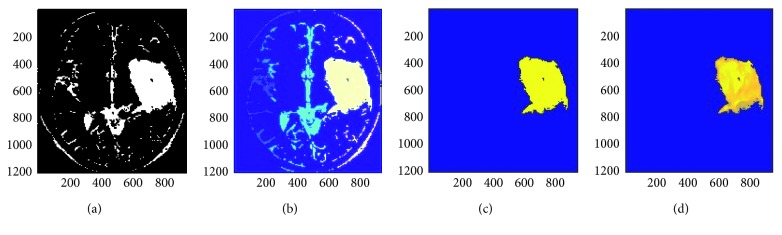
Tumor in brain region segmentation in a sample tumor brain MRI image. The brain MRI image after performing fuzzy *c*-means and connected regions operations is shown along with the final segmented tumor region and mask using the fully automatic procedure for tumor segmentation from the brain segmentation. This shows that the method proposed in this paper successfully works for tumor segmentation and identification along with gray and white matter segmentation. Thus, brain tumor segmentation is another application of this paper's proposed algorithm along with gray and white matter region segmentation. (a) Fuzzy tumor region. (b) Connected tumor region. (c) Segmented tumor region. (d) Tumor region mask.
